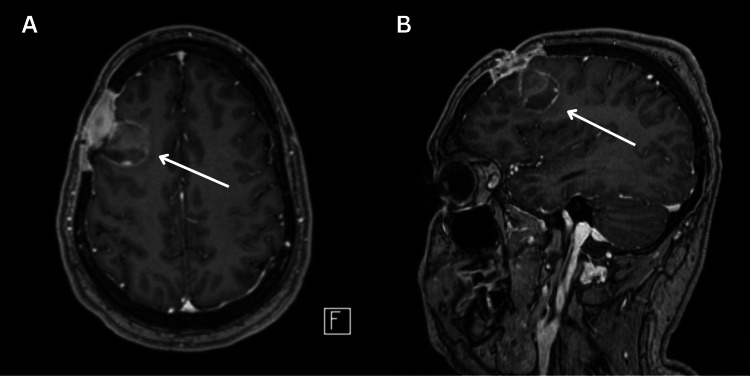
Head imaging at the three-month follow-up Axial (A) and sagittal (B) view of a post-gadolinium T1-weighted brain MRI at the three-month follow-up showing a small (3.0 x 3.4 cm) rim-enhancing collection within the right frontal lobe with internal diffusion restriction, which is likely related to hemorrhagic products.
